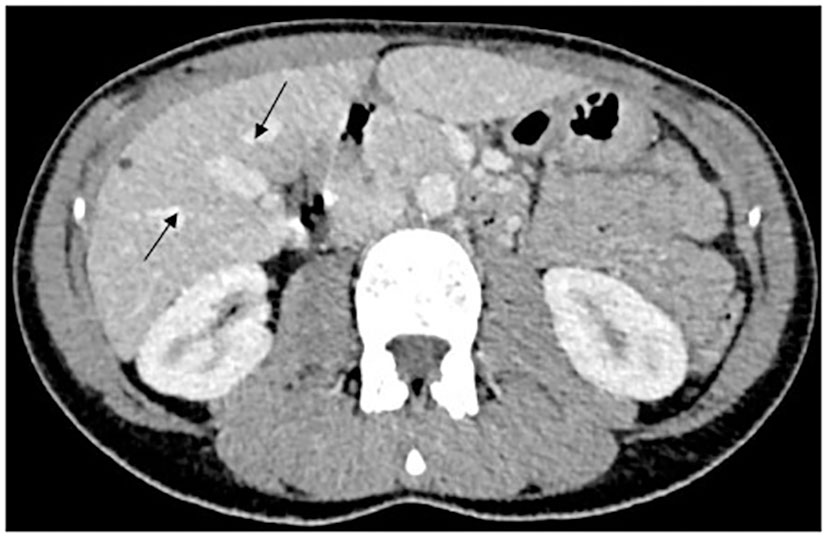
MRI hyper signal T2 showed two focal lesions of segments VIII and VI, and liver metastases from CM.
